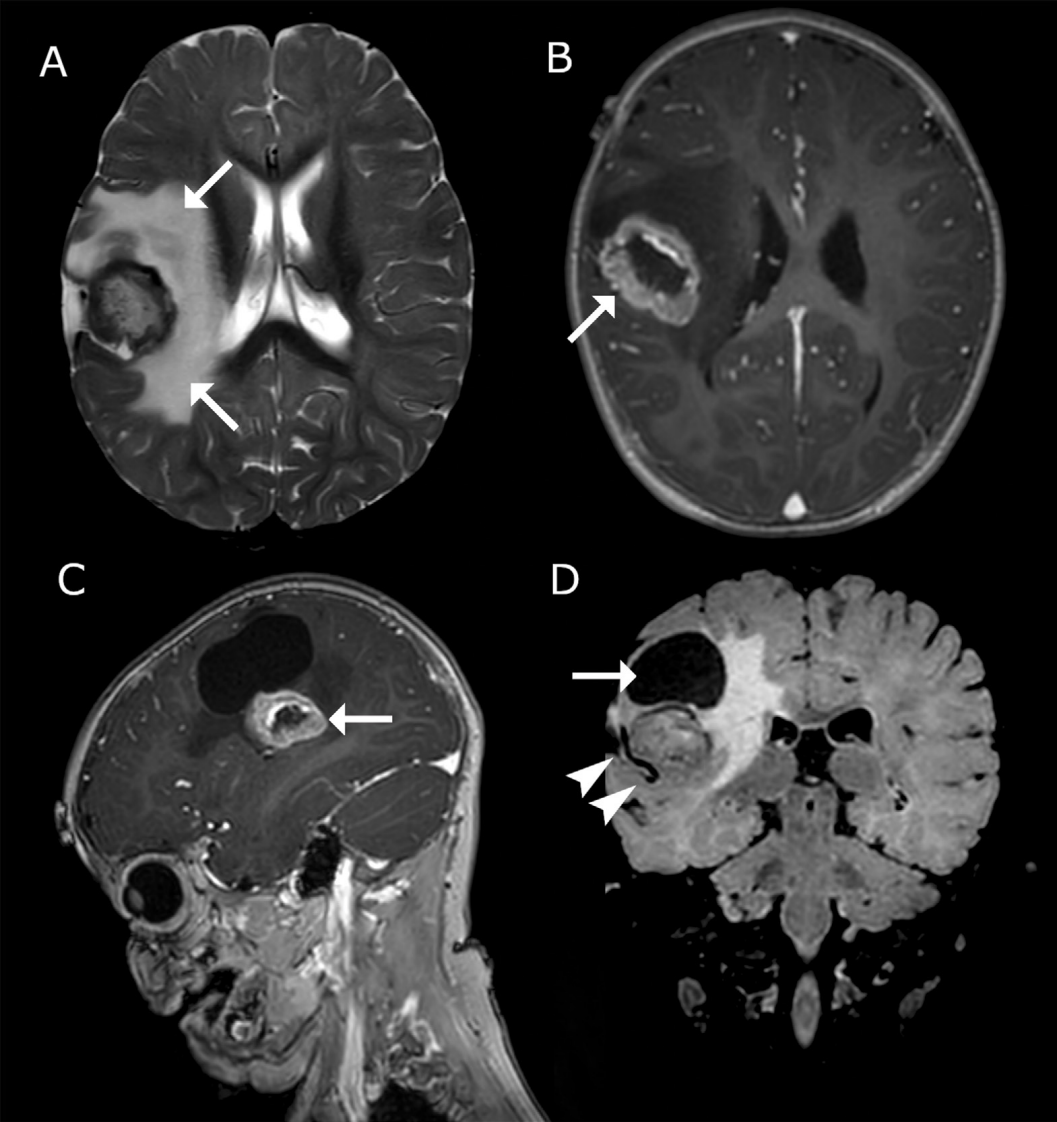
Imaging findings on 3‑Tesla MRI brain. (A) Axial T2‑wi showing a cortically based lesion in the right cerebral hemisphere with perilesional edema (arrows). (B&C) 3D T1‑wi; the peripheral, solid components show contrast‑enhancement (arrows). (D) FLAIR; coronal images demonstrate a large peritumoral cyst (arrow). In addition, a flow void of an MCA branch is seen traversing the lesion (arrowheads), in keeping with encasement.
